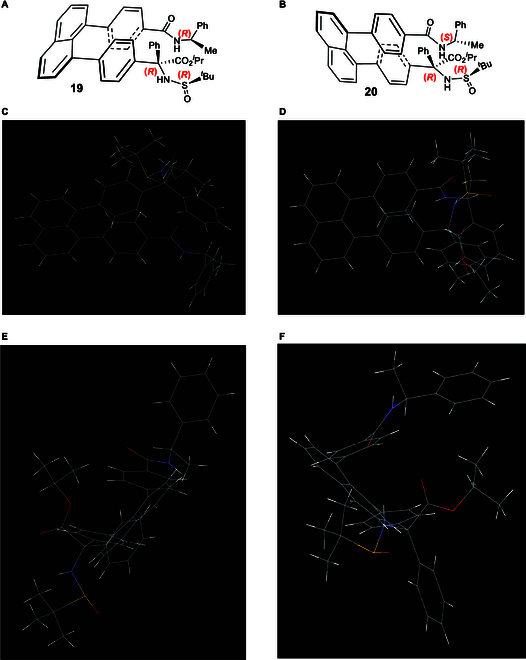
Chiral staircase targets 19 and 20 and their x-ray structural analysis. (A and B) Chemical structures of two staircase isomers. (C and D) Views of their x-ray structures from top to bottom. (E and F) Views of their x-ray structures from side to side.
